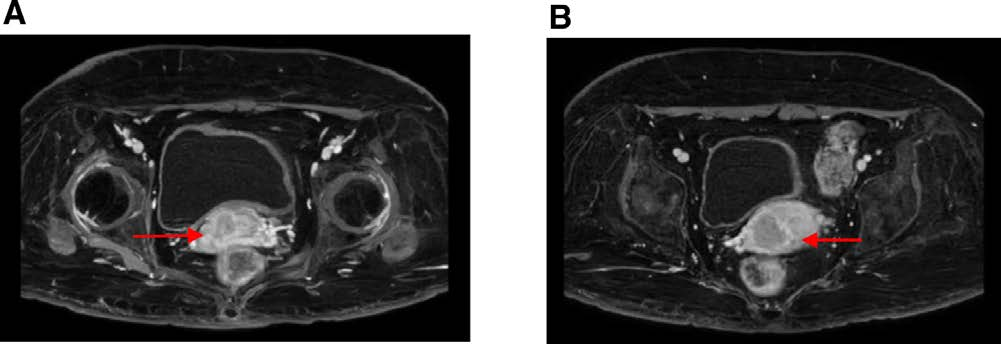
Pelvic contrast-enhanced MRI, showing the presence of uneven enhancement mass (arrow) in the right part of the cervix(A) and the posterior part of the uterine body(B), some parts of the cervical stroma are discontinuous, and the parauterine space on both sides is clear (before radiotherapy for CC). CC = cervical cancer, MRI = magnetic resonance imaging.
